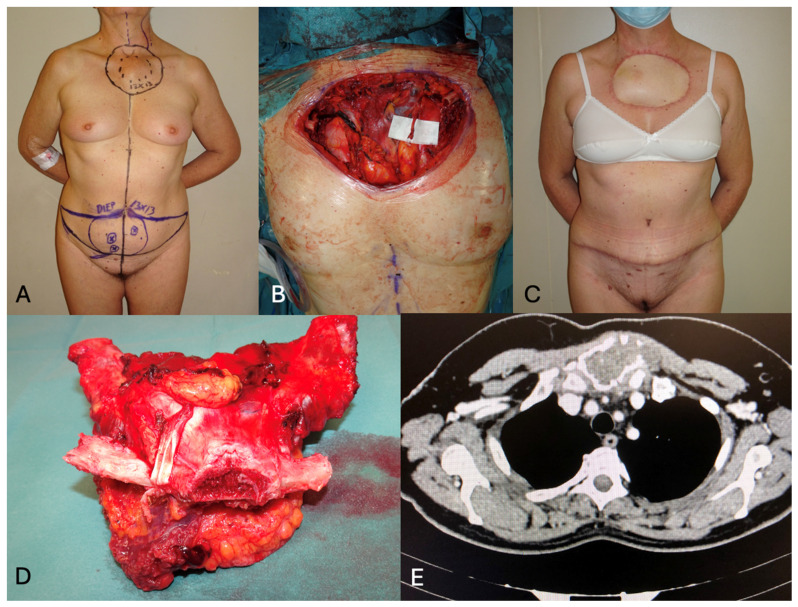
(A) a case of chondrosarcoma involving the sternal manubrium and the first 2 ribs bilaterally in a 60-year-old female patient. The extensive defect measured 13 × 13 cm. The patient was scheduled for DIEP flap reconstruction, which was the only available donor site to allow primary closure in an aesthetically acceptable fashion in the supine operative position. (B) Intra-operative view of the extensive defect with exposure of both the lungs and aortic arch. The left internal mammary vessels were prepared for anastomoses. (C) Six-month post-operative view showing a well-settled flap with aesthetic improvement of the abdominal contour after closure in an abdominoplasty fashion. (D) Excised tumor. (E) Pre-operative CT showing the degree of local infiltration.
